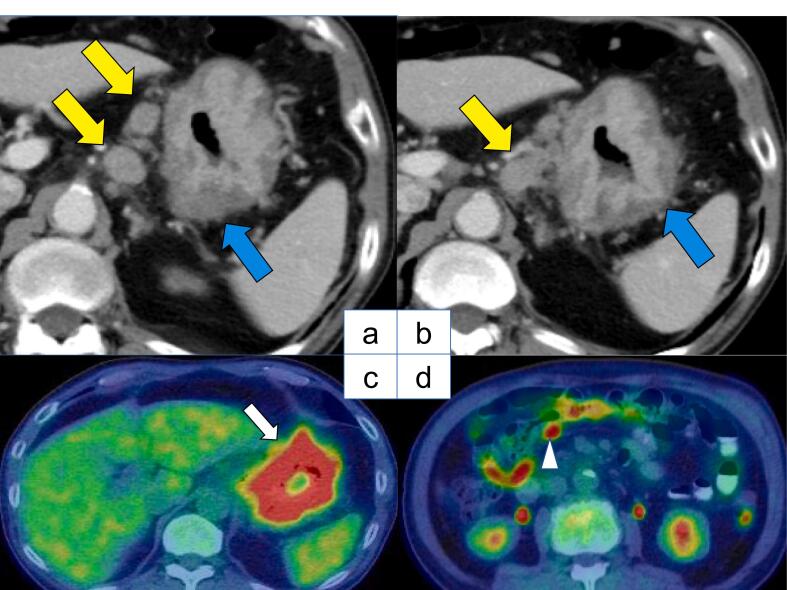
a, b - Images of pretreatment abdominal computed tomography (CT). The blue arrow shows irregular gastric wall thickening on esophagogastroduodenoscopy, consistent with a neoplastic lesion. The yellow arrow shows the enlarged lymph nodes of the lesser curvature. c - Images of pretreatment positron emission tomography (PET)–CT images. The white arrow shows uptake along the cardia to the greater curvature of the gastric region. d - The white arrowhead shows the peritoneal nodule demonstrating high fluorodeoxyglucose (FDG) uptake. (For interpretation of the references to color in this figure legend, the reader is referred to the web version of this article.)
